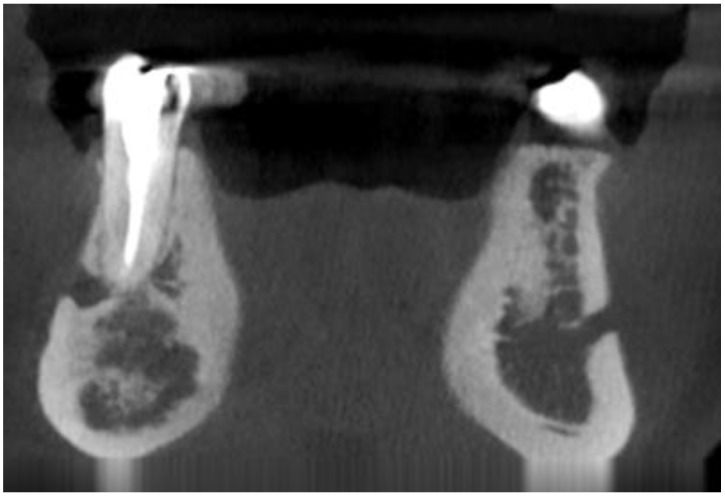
CBCT-coronal view, COD in the premolar area in the right mandibular basis.
